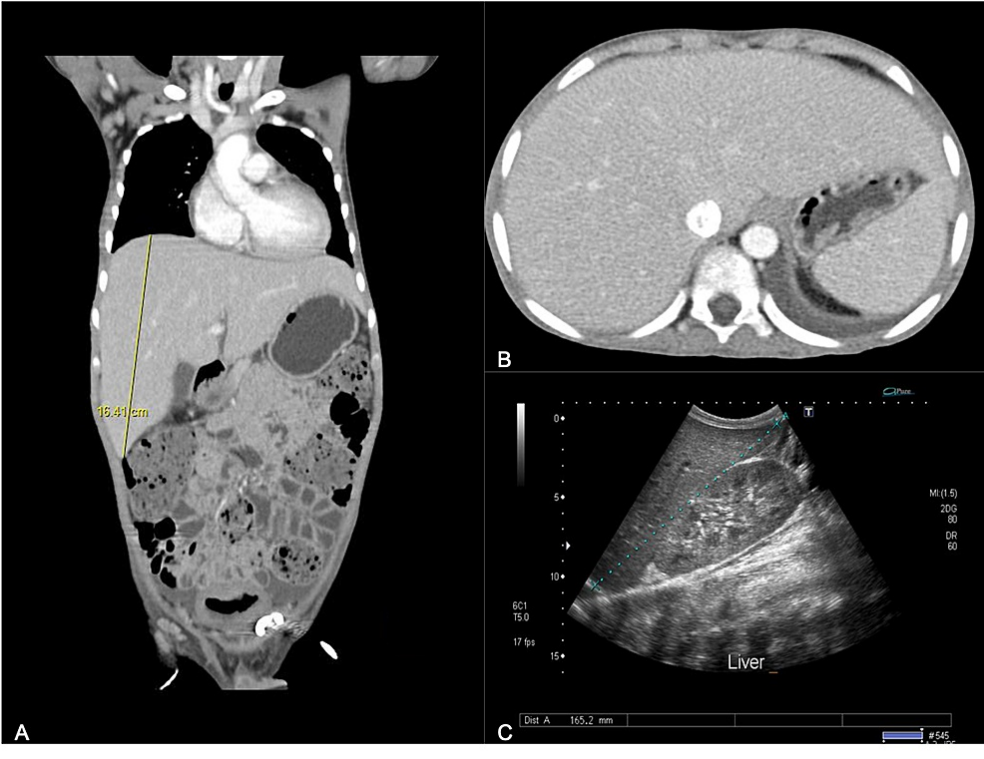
Abdominal imaging of patient in case 1 (A) Coronal CT scan view of the abdomen shows an enlarged liver. (B) Transverse view shows hepatomegaly, peri-portal edema, and moderate ascites with suspected intra-abdominal collections. (C) Longitudinal gray scale ultrasound view shows an enlarged liver measuring 17.3 cm.
